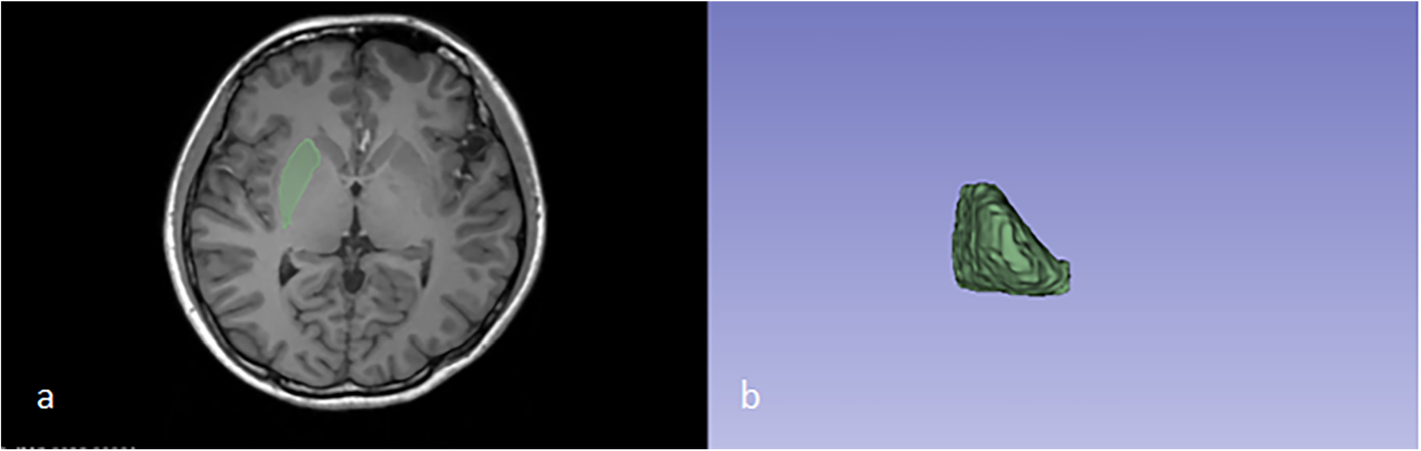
The process of segmentation. a VOIs were drawn in all axial MRI images. b VOI masks extracted from MRI scans
